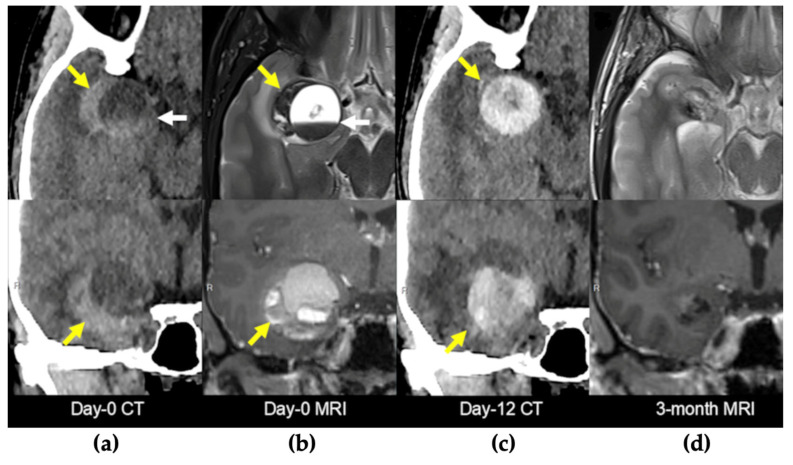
Neuroradiological findings. The upper row displays axial sequences, while the lower row shows coronal sequences. In (b,d), the upper row corresponds to a T2-weighted MRI, and the lower row to a contrast-enhanced T1-weighted MRI. (a,b) CT scan and MRI scan obtained at the patient’s initial admission showing a right temporopolar hematoma (yellow arrow) due to the rupture of a cavernoma containing a hemato-hematic level (white arrow). (c) CT scan performed upon readmission, 12 days after discharge, demonstrated new acute bleeding within the lesion (yellow arrow). (d) MRI performed three months after surgery showed small area of fibrotic tissue with no evidence of residual lesion or ischemic areas. CT: computed tomography; MRI: magnetic resonance imaging.
